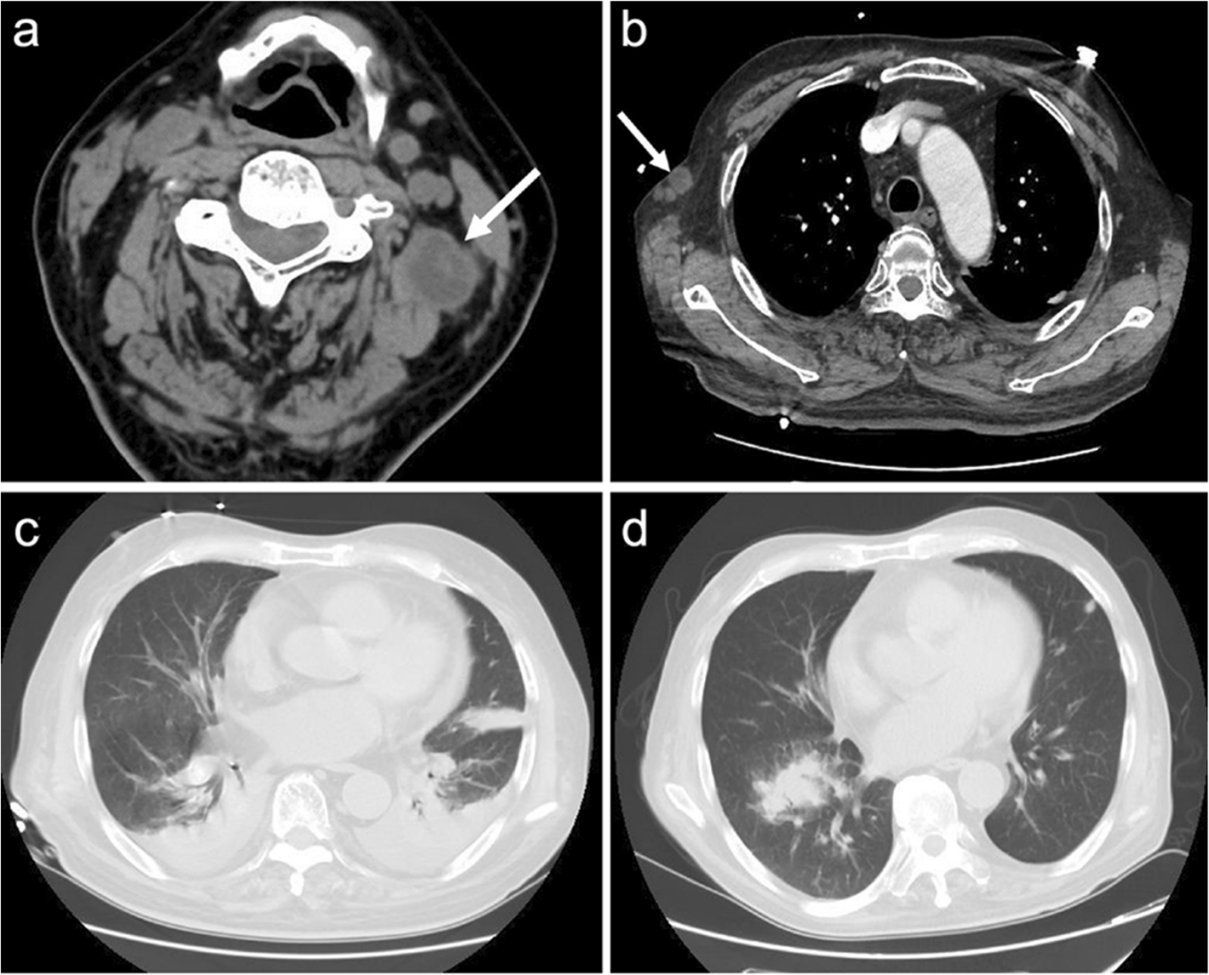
CT images of the neck and chest on the day of admission to hospital (a, b, c): a CT scan showing a mass on the left of the neck, arrow shown in (a). CT of the chest showing the subcutaneous nodes, with the arrow shown in (b). There were multiple nodular or patchy shadows in the bilateral lungs and pleural and pericardial effusion (c). One month after treatment with SMZ-TMP, a repeated chest CT (d) showed pleural effusion and that the lesions in the left lung had disappeared while the lesions in the right lung had become more apparent
